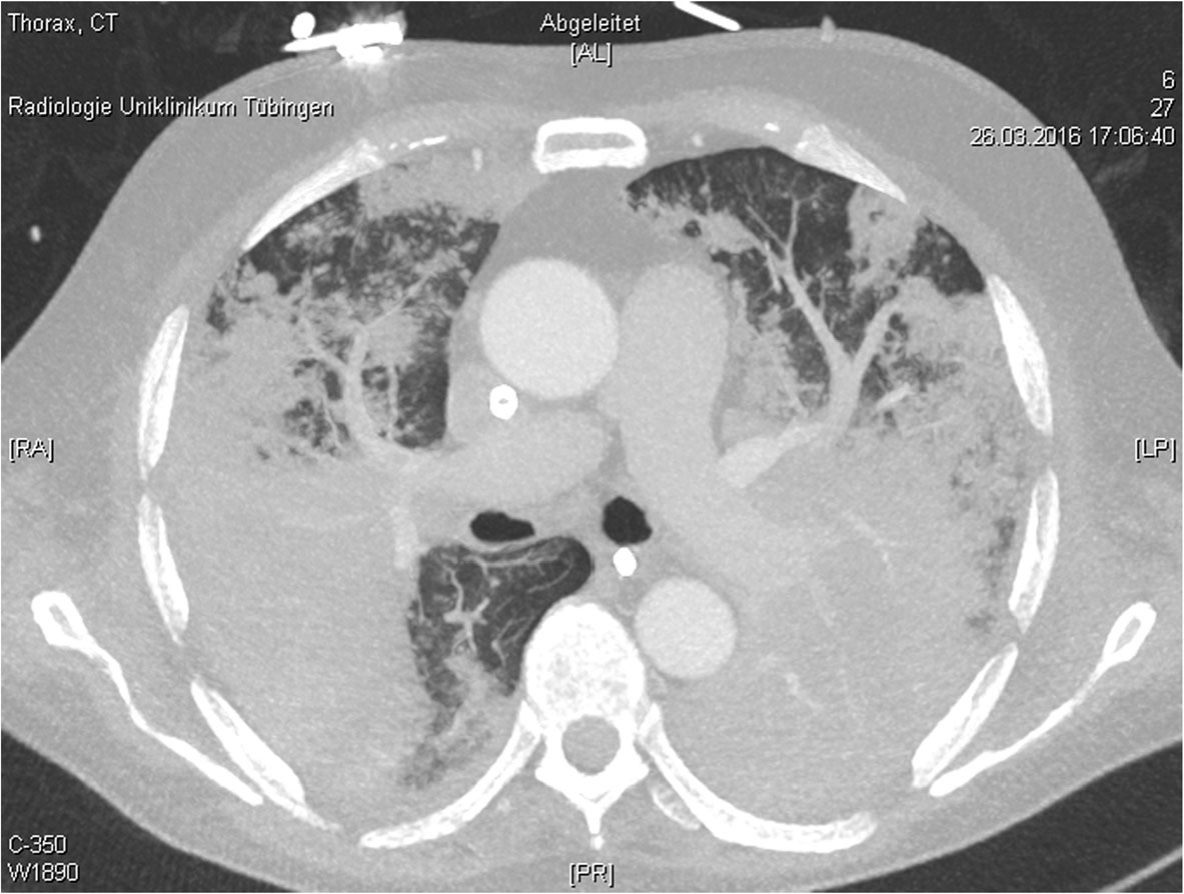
CT scan of the lung on day 12 after admission
